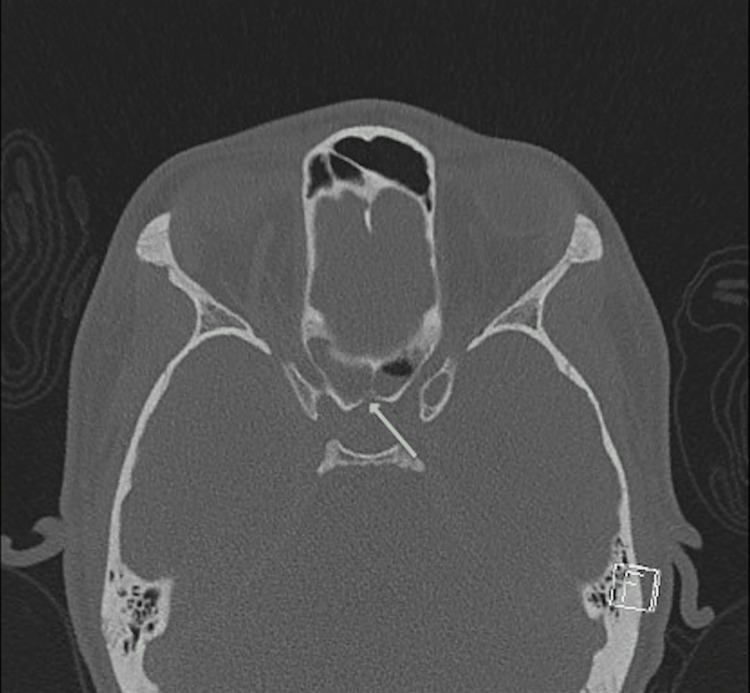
CT scan of sinus with contrast, axial cut, ethmoid and sphenoid sinus disease with bony dehiscence along posterior wall of right sphenoid sinus/anterior sella (arrow) CT, computed tomography.
